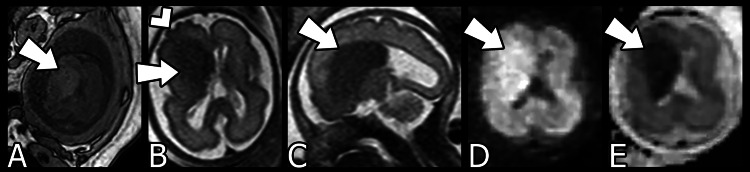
Fetal MRI at 26 weeks’ gestation A) Axial T1-weighted, B) Axial T2-weighted, C) Sagittal T2-weighted, D) Diffusion-weighted imaging (DWI), E) Apparent diffusion coefficient (ADC). The arrows demonstrate a mass-like focus in the region of the right frontal germinal matrix with increased signal on T1-weighted imaging and decreased signal on T2-weighted imaging. Additionally, there is associated restricted diffusion as evidenced by increased signal on DWI and decreased signal on ADC. The arrowhead indicates cortical irregularity along the right frontal lobe suspicious for polymicrogyria.
